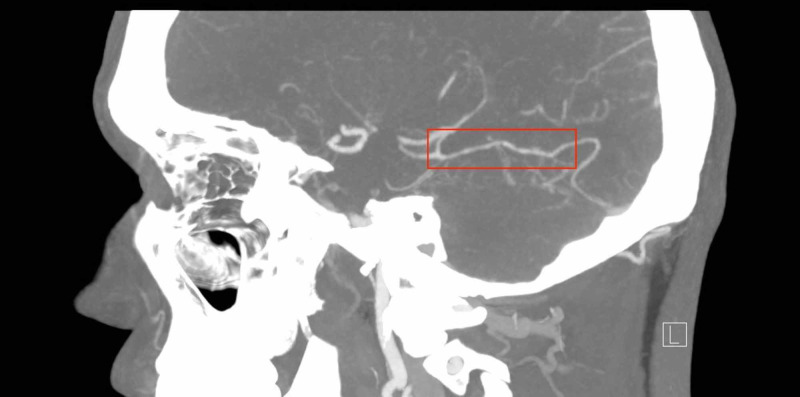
Sagittal view of the "string of beads" appearance indicative of vasospasm, as seen on CTA. CTA, CT angiogram
